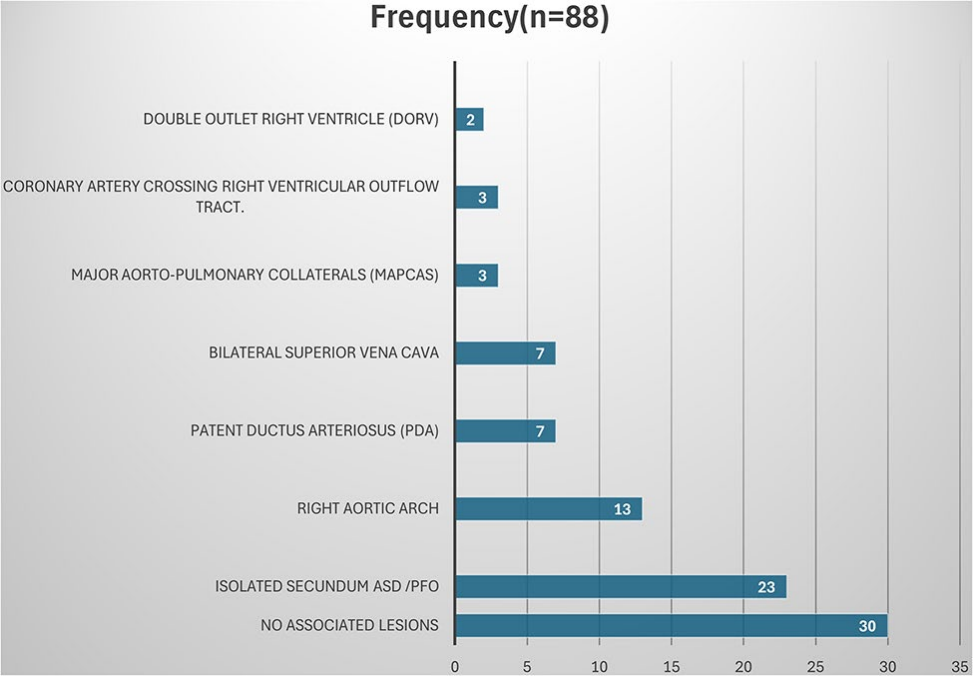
Echocardiographic findings of associated cardiac defects amongst patients who underwent primary TOF repair at UHI. *ASD = atrial septal defect, PFO = patent foramen oval*
